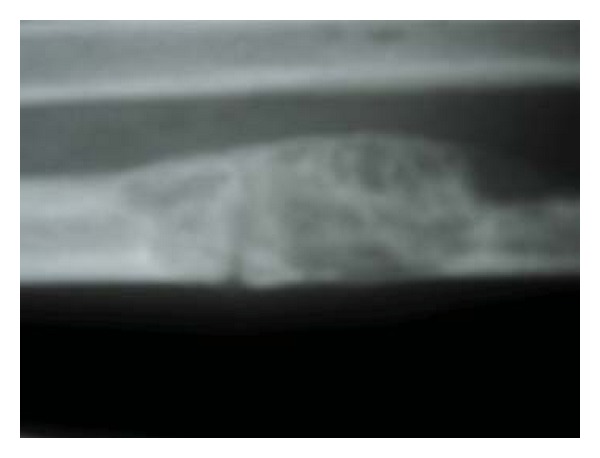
A lateral X-ray of the right ulna showing healing of the pathologic fracture in progress (8 weeks after curettage).
